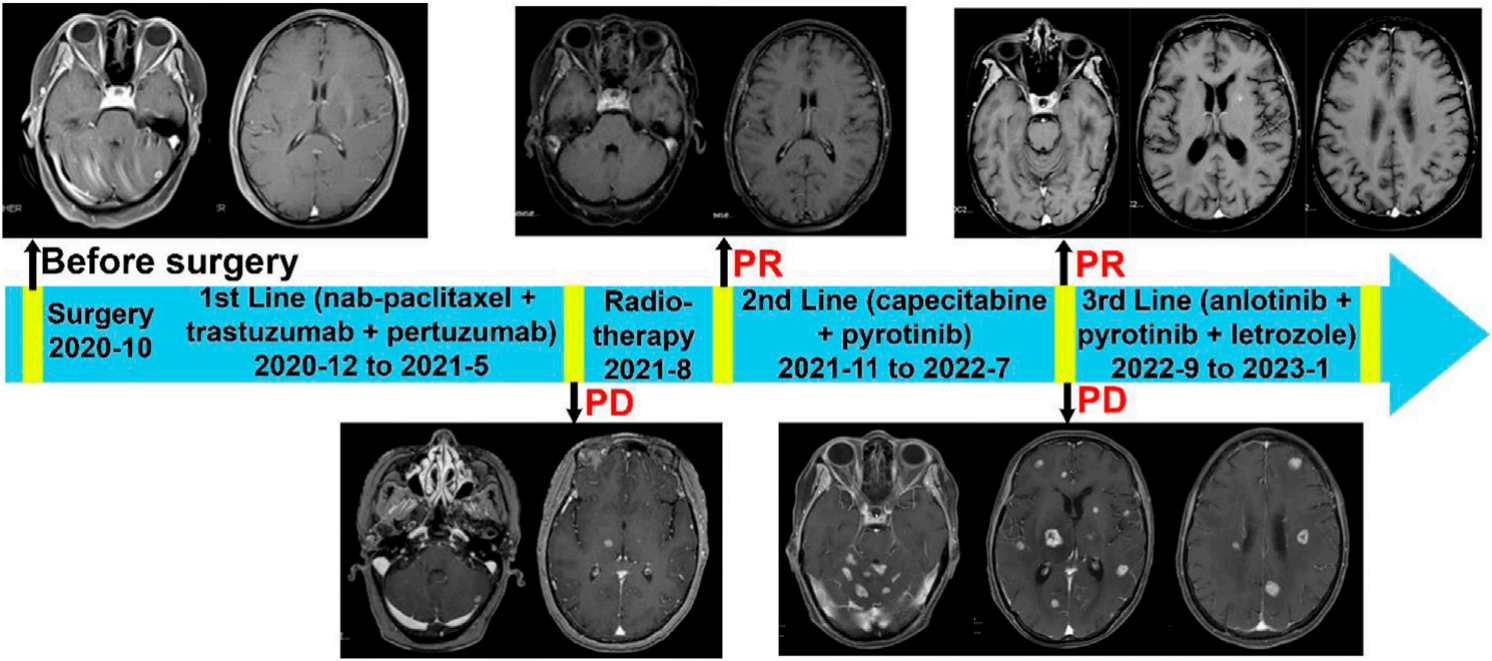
Complete treatment process of the patient since diagnosis. Brain MRIs of the patient were displayed. 1) Enhanced MRI of the head upon patient’s admission in December 2020 displays multiple intracranial metastatic tumors. 2) The enhanced MRI of the head from August 2021 shows that compared to the baseline scan, the size and number of intracranial metastatic foci have increased (prior to radiation therapy). 3) Follow-up enhanced MRI of the head after radiation therapy in September 2021 demonstrates a reduction in the number and size of the intracranial metastatic tumors. 4) Before the Anlotinib treatment, the enhanced MRI of the head in August 2022 shows that the size and number of multiple brain parenchyma metastatic tumors have increased compared to previous scans. 5) The follow-up enhanced MRI of the head in November 2022 shows a significant reduction in the number and size of metastatic tumor lesions compared to previous scans.
